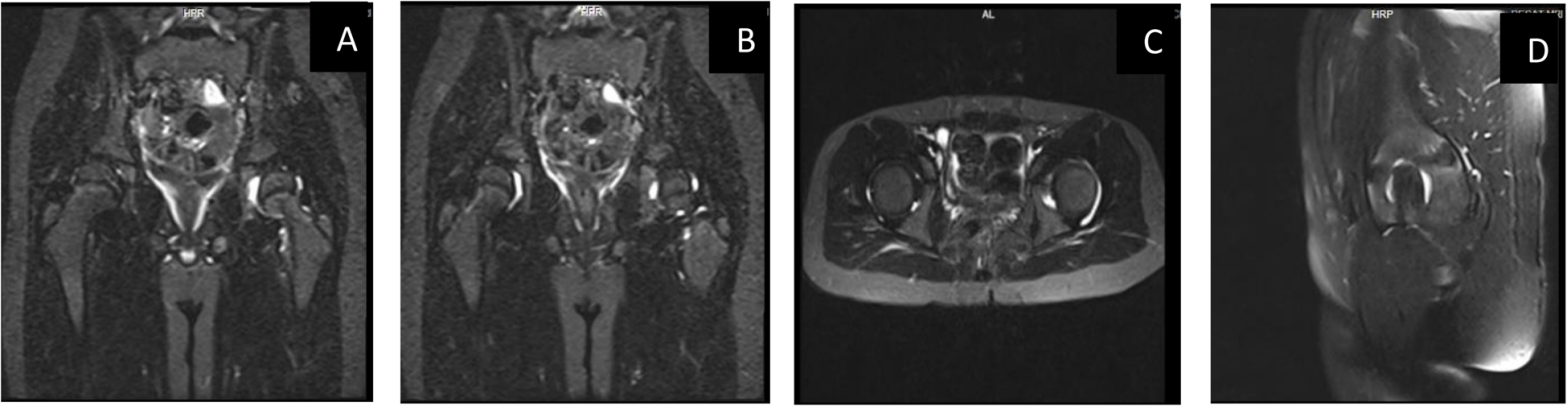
STIR sequencing MRI of both hip joints of the first patients. a-b Coronal view. c Axial view. d Sagittal view. Both hip joints effusion, especially in left side
